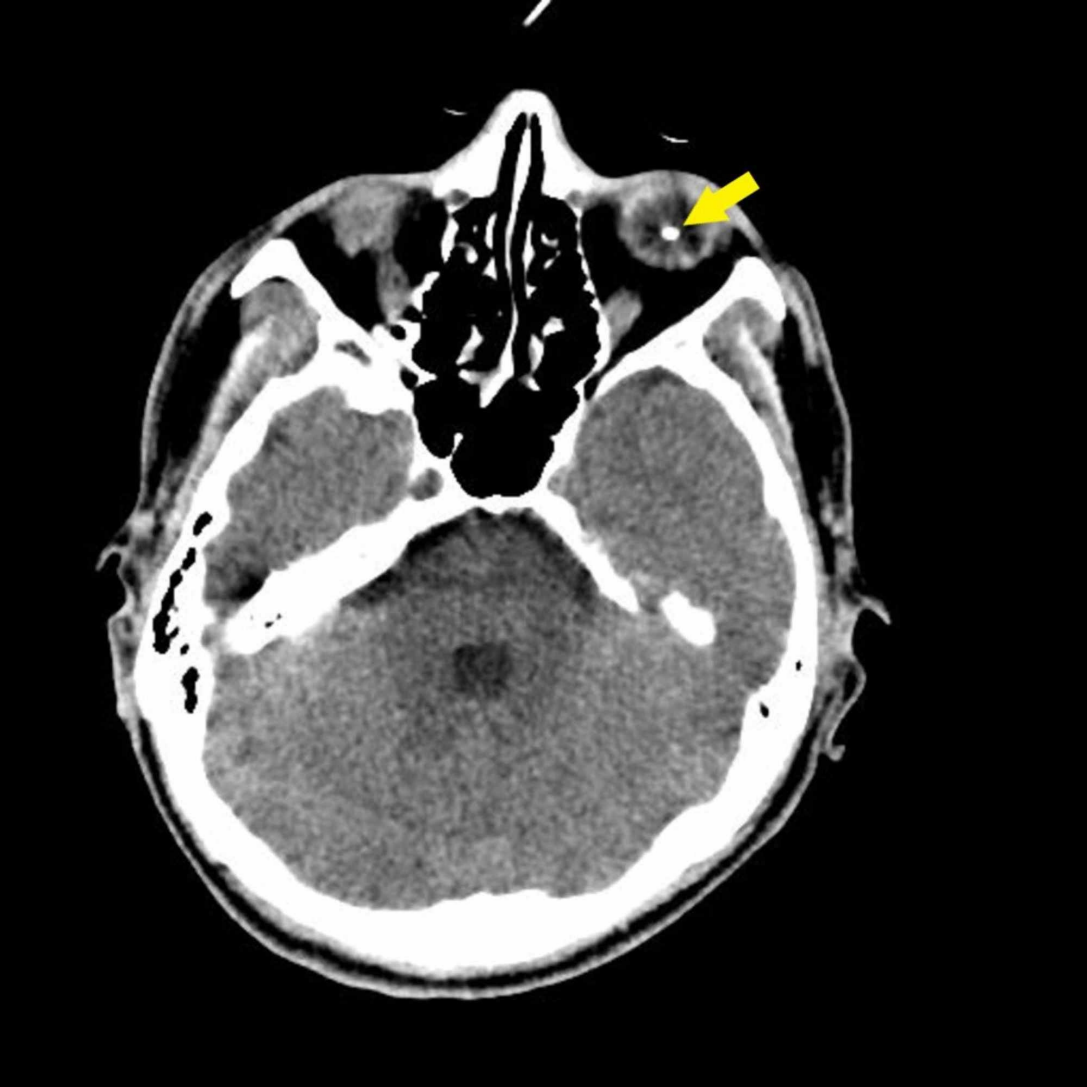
Axial view of the plain CT scan of the brain in this patient, showing a 0.5 cm diameter hyperdense object (arrow), with streak artifacts in the inferior left globe.
